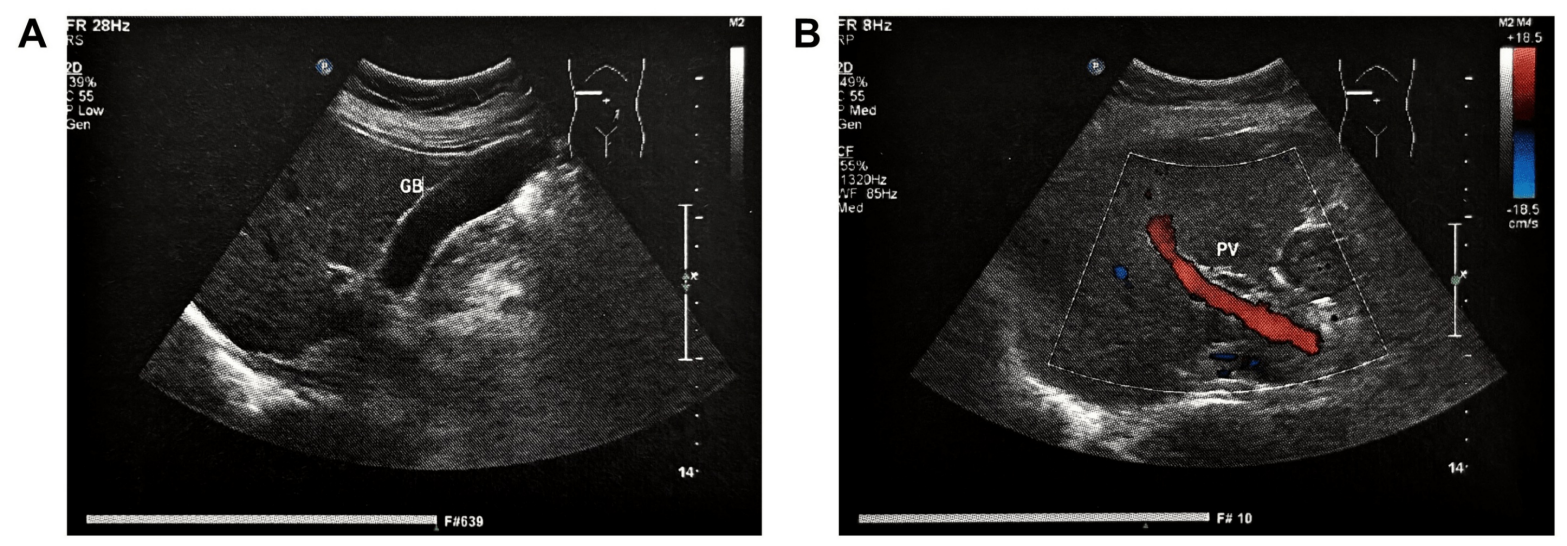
Hepatobiliary-pancreatic-splenic and portal venous color Doppler ultrasonography in December 2023 (22 weeks after lesion resolution and consolidation follow-up of compensating bio-information energy (CBE) modulation).
